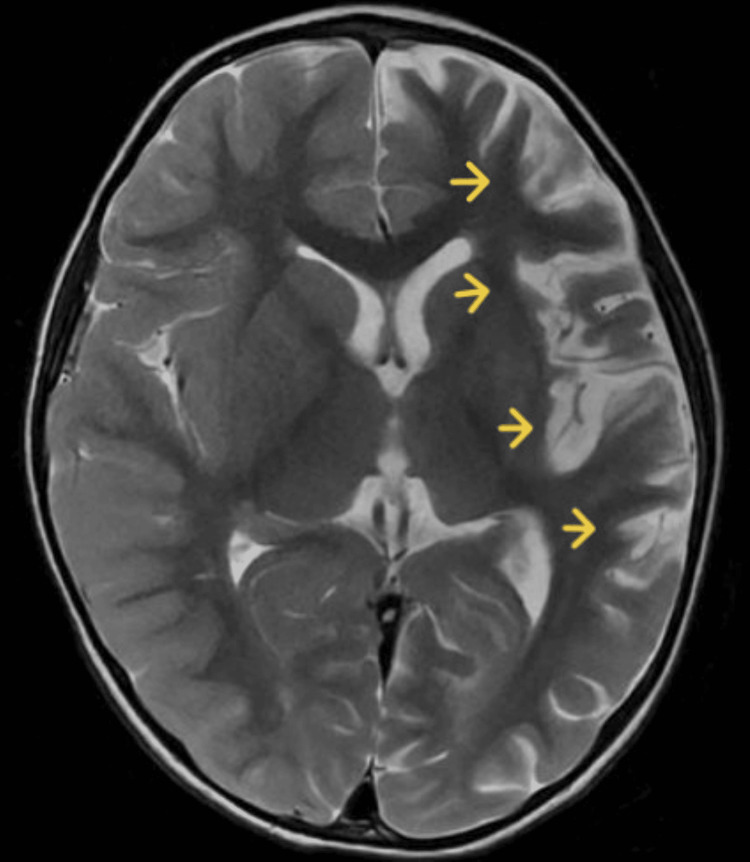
First brain MRI axial T2
